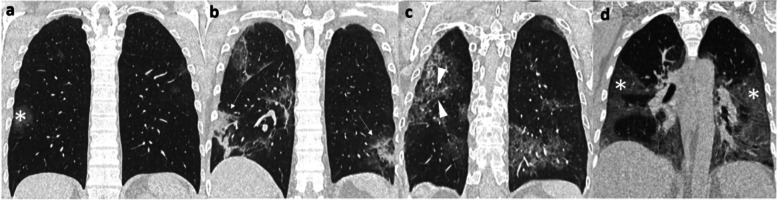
Coronal chest CT images representing the visual scoring system used to evaluate the degree of parenchymal involvement: < 20% (a), 20–39% (b), 40–59% (c), > = 60% (d). Parenchymal qualitative CT findings evaluated in this study were: ground-glass opacities (asterisks in panels a and d), consolidation (arrows in panel b), crazy-paving pattern (arrowheads in panel c)
